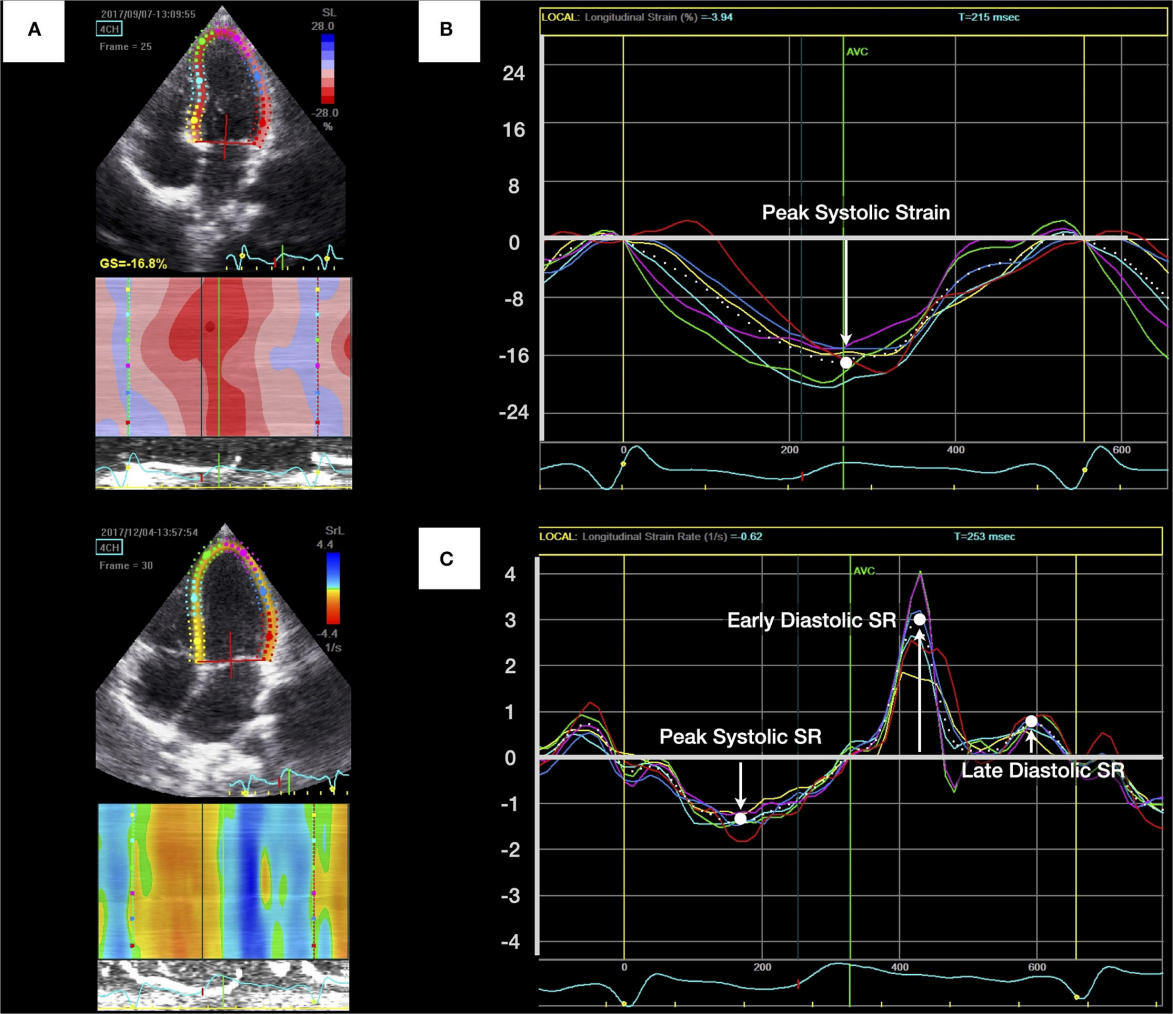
Measurement of left ventricular (LV) longitudinal peak systolic strain and strain rate (SR). (A) Apical four-chamber view of the left ventricle with the region of interest. (B) LV longitudinal peak systolic strain was identified as the highest point and calculated by averaging measurements of six curves. (C) The peak systolic SR, early diastolic SR, and late diastolic SR were generated from LV apical four-chamber views.
